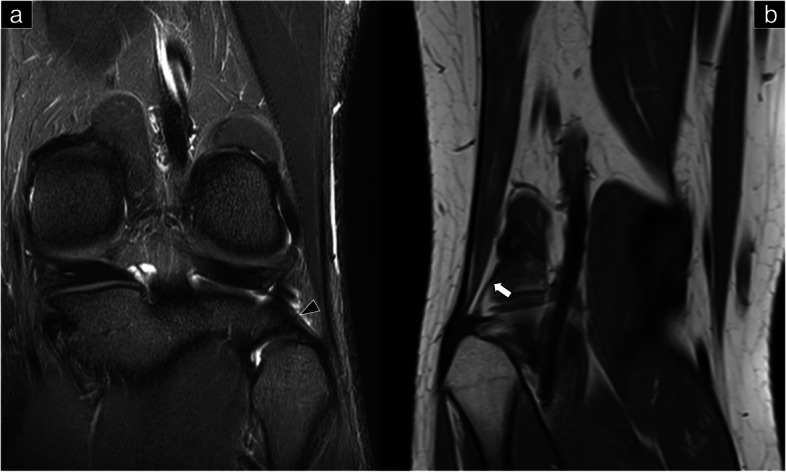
Magnetic resonance imaging shows the popliteofibular ligament (a) and the fabellofibular ligament in the coronal plane (b). Black arrowhead: popliteofibular ligament; white arrow: fabellofibular ligament
